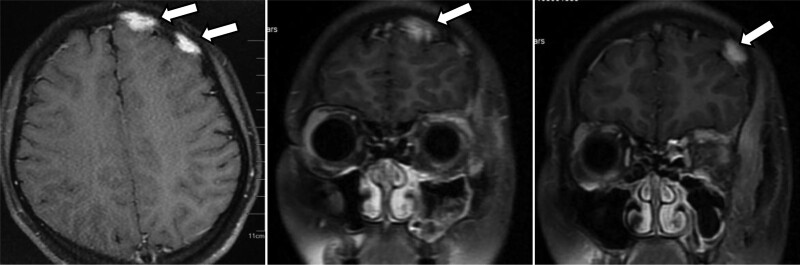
MRI showed two patchy isosignal foci on the left side of frontal bone, with sizes of 2.5 cm*1.1 cm and 1.8 cm*0.8 cm, respectively. The enhanced scan showed obvious enhancement. MRI = magnetic resonance imaging.
